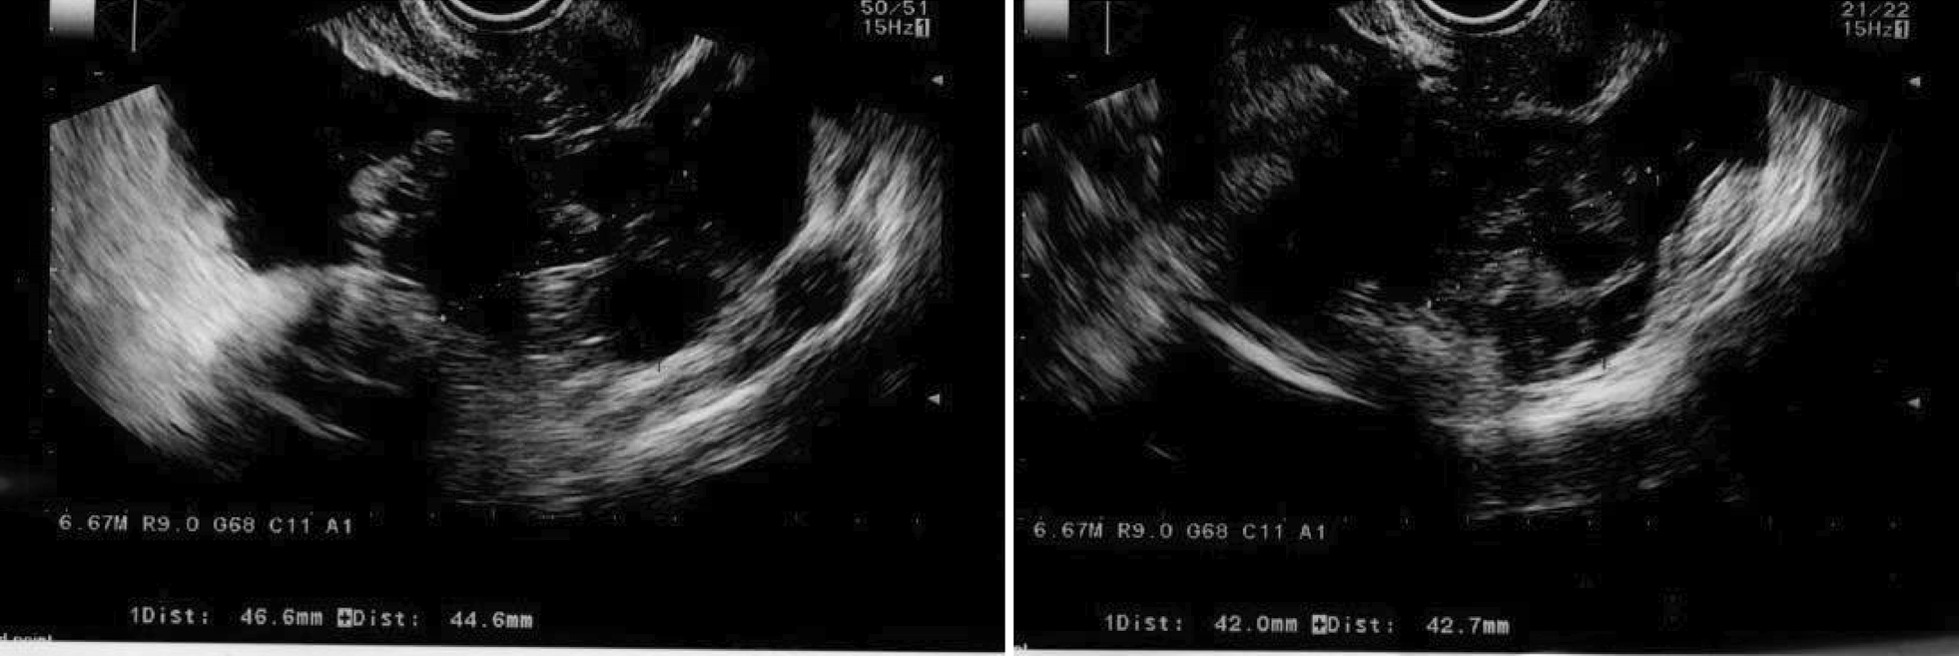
Ovarian ultrasonography before discharge, showing bilateral shrinkage of the ovarian swelling to ~ 4.5 cm size
